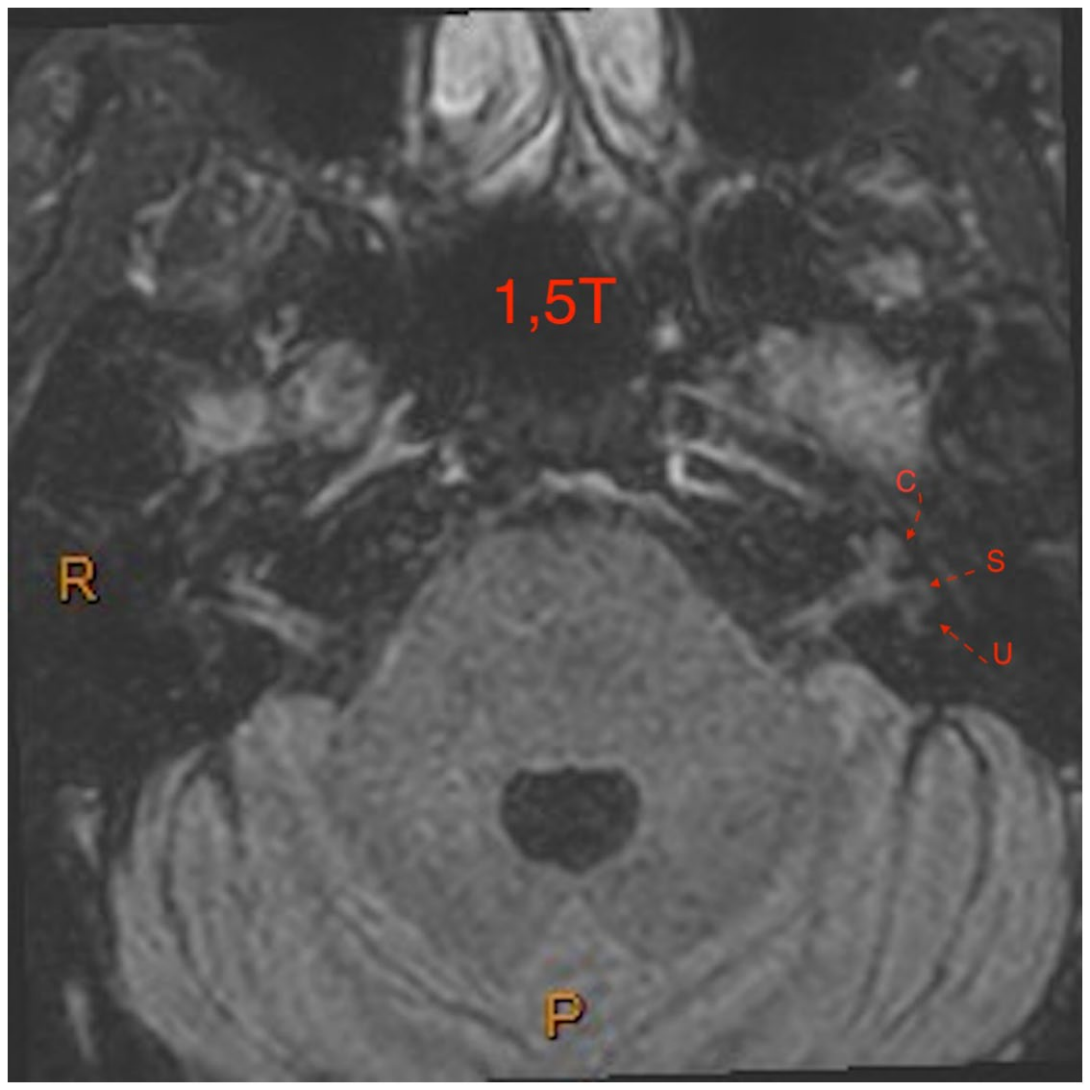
1.5 T MRI axial scan, sequence 3D-FLAIR, left ear with grade 0 of Barath in cochlea and vestibule.
